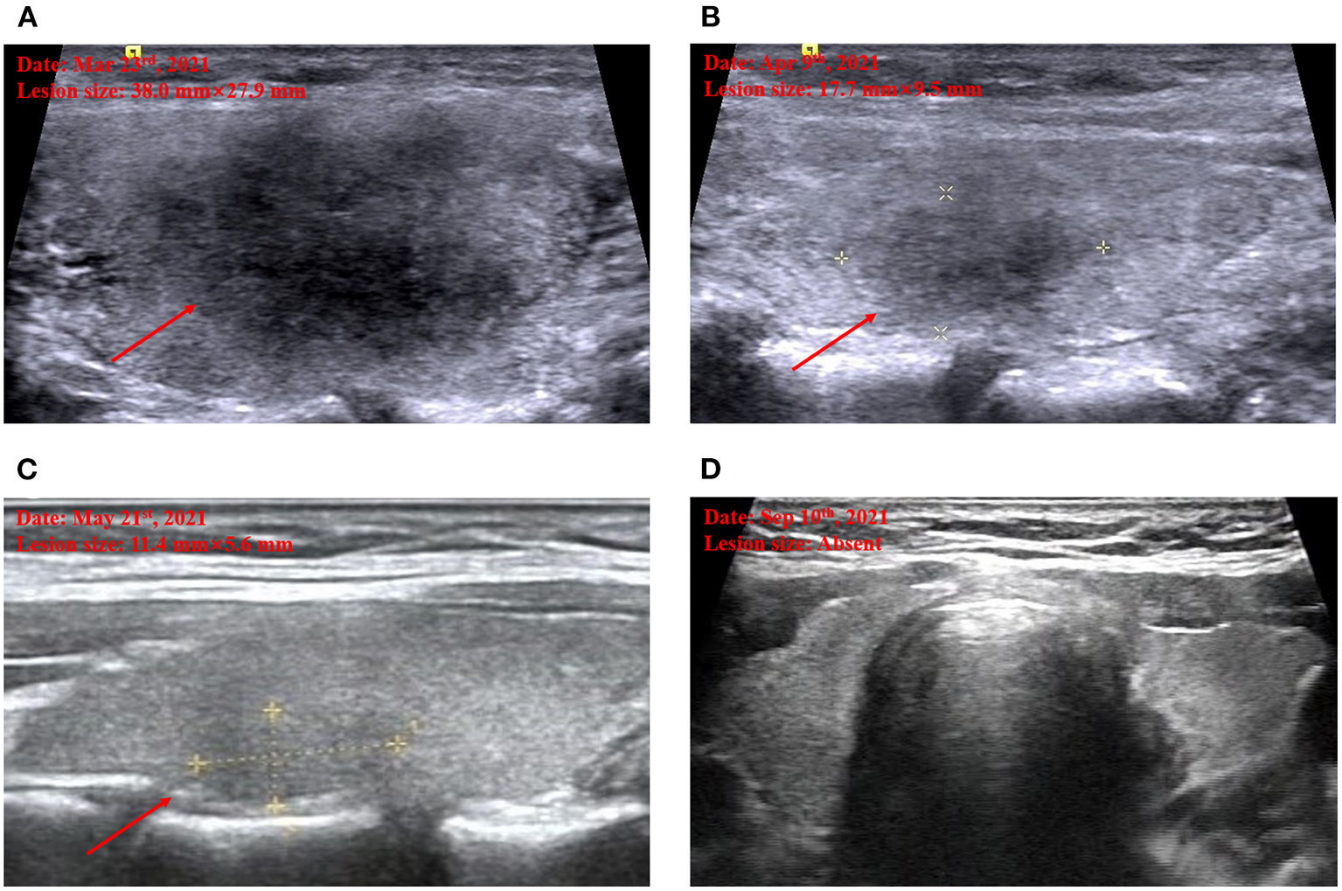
Thyroid ultrasound imaging. Marked hypoechoic lesion on admission (A). Decreased hypoechoic lesion by half when the first follow-up on April 9 (B). Further decreased hypoechoic lesion when the second follow-up on May 21 (C). Disappeared hypoechoic lesion when the third follow-up on September 10 (D).
